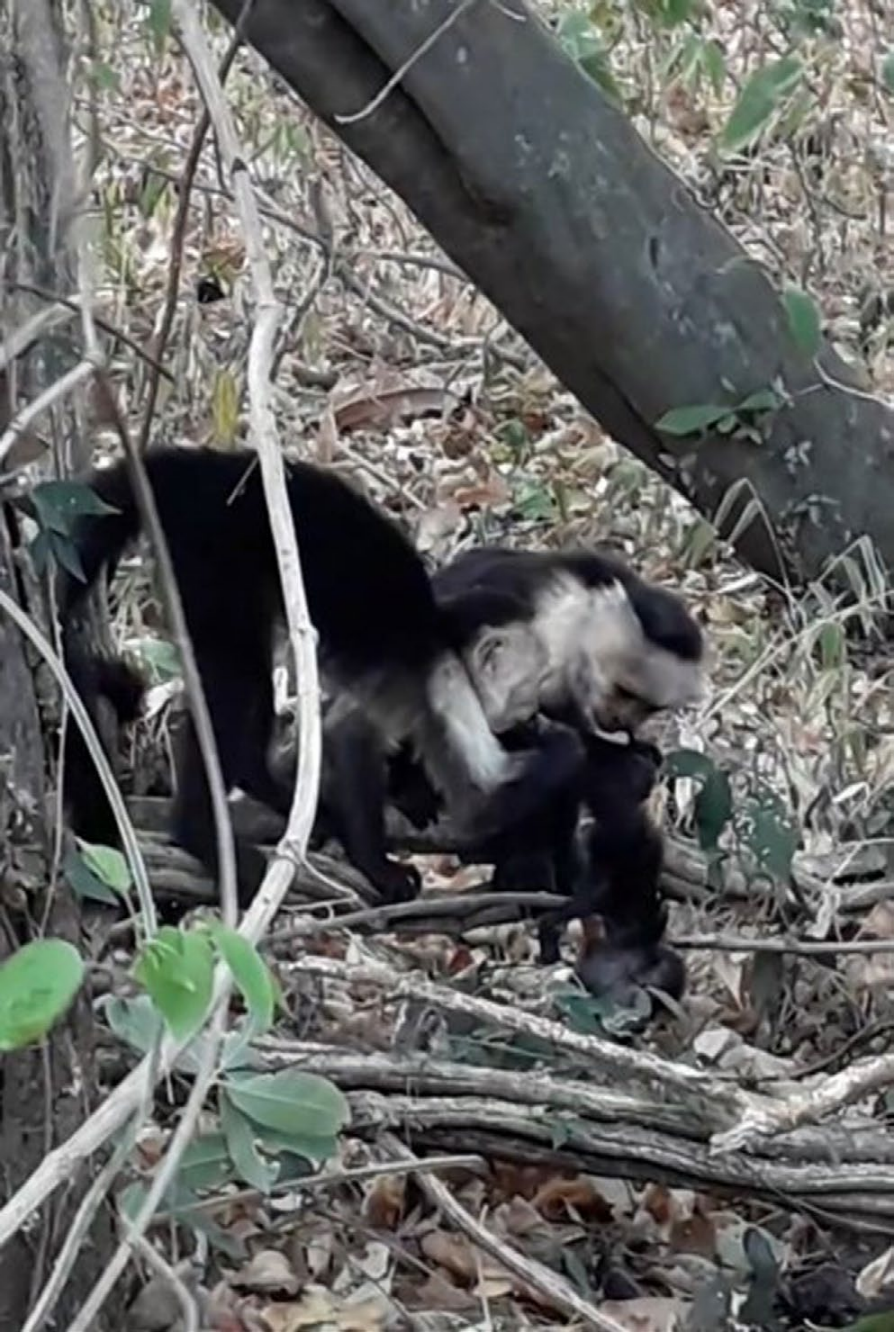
Adult female (right, SS) and juvenile male (left, BS) holding and eating the dead infant (CT‐19) (Photograph by N. Ferrero)
